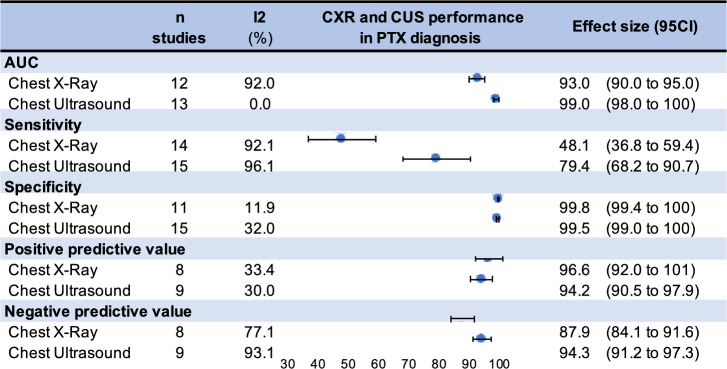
Summary of all meta-analyses for Chest X-Ray (CXR) and Chest Ultrasound (CUS) performance diagnosis in pneumothorax (PTX) for area under the curve (AUC), sensitivity, specificity, positive predictive value (PPV), negative predictive value. Blue dots represent the overall effect size for CXR and CUS in area under the curve (AUC), sensitivity, specificity, positive predictive value (PPV), negative predictive value. The lenght of each horizontal line around the dots represents their 95% confidence interval (95CI). I-squared (%): percentage of heterogeneity between studies for each meta-analysis
